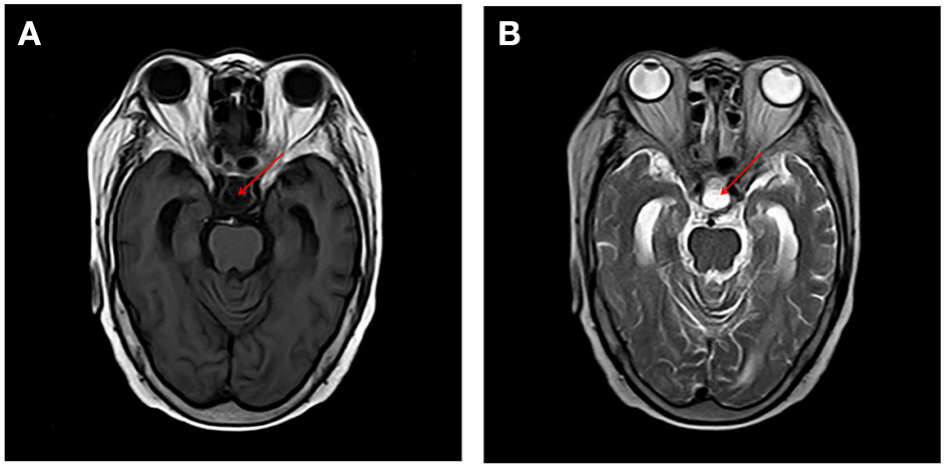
MRI of the brain revealed change after removal of pituitary tumor and no acute cerebral infarction, cerebral hemorrhage, and inflammatory changes were found on T1 and T2 sequences [T1, T1-Weighted Magnetic Resonance Imaging; T2, T2-Weighted Magnetic Resonance Imaging (A) T1, (B) T2].
